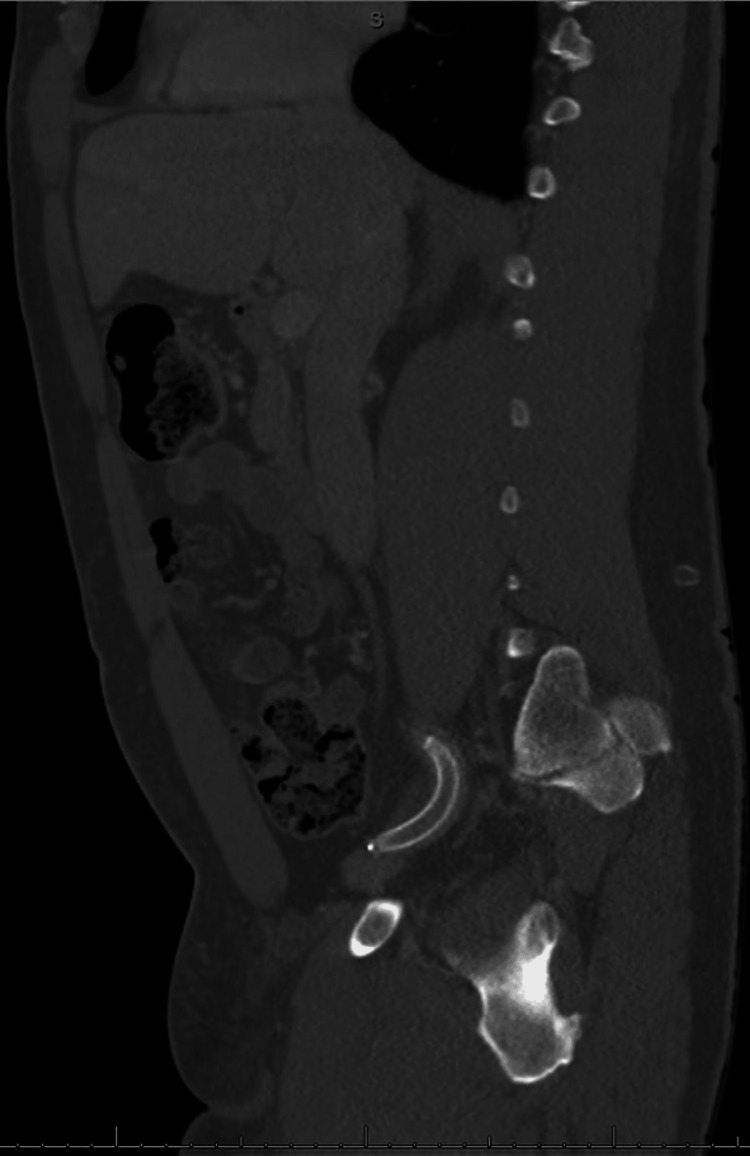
Computed tomography angiography demonstrating right external iliac stent
